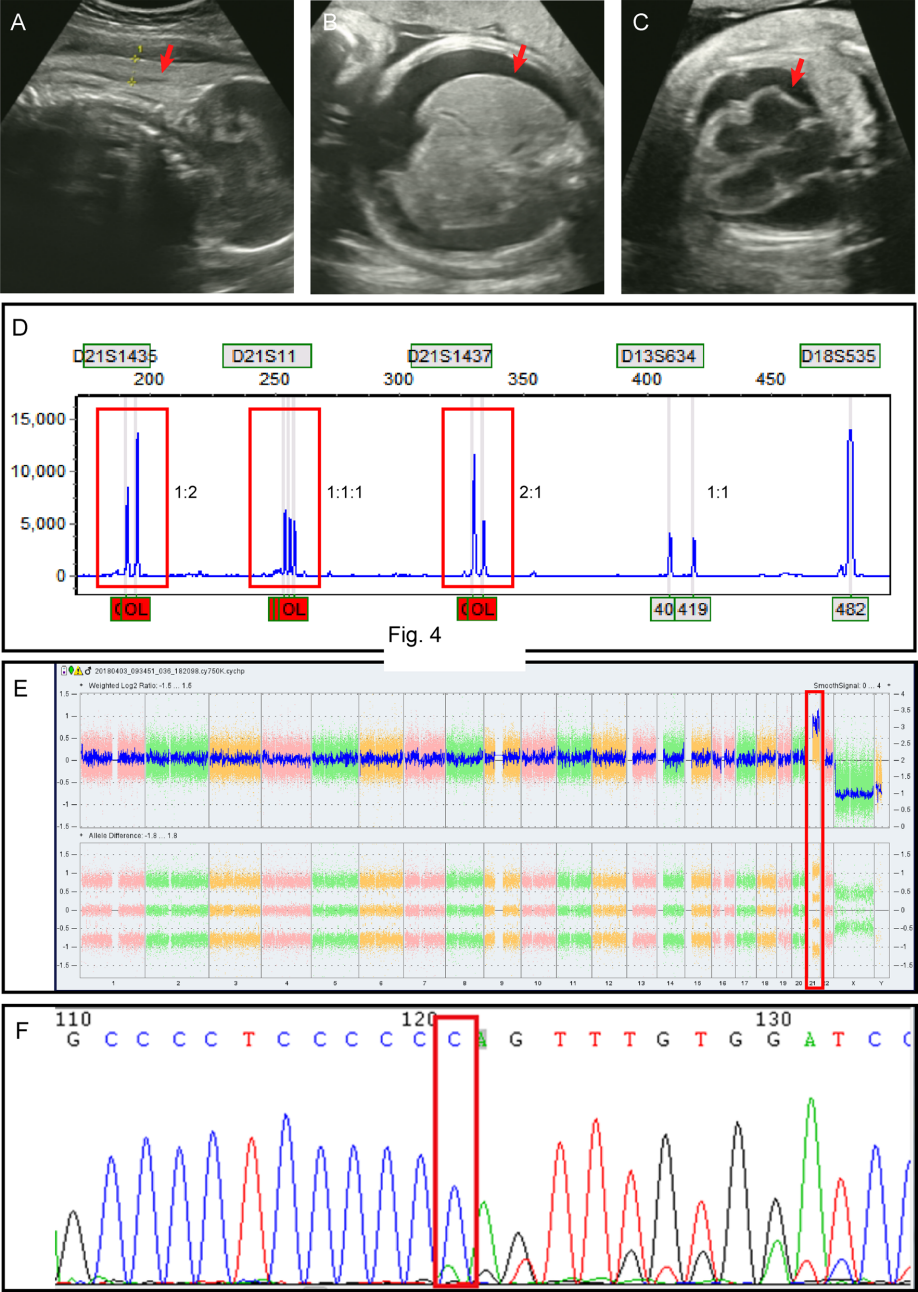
Case2 A–C: The 31-week ultrasound findings of the foetus. A The foetus had thickened skin on the head and chest. B Foetal ascites. C Foetal heart enlargement combined with pericardial effusion. D Electrophoretograms of multiplex QF-PCR amplification with STR markers on chromosomes 18, 13 and 21. Electrophoretic profiles observed for trisomy 21 foetus (red boxes). E Image from the Affymetrix Chromosome Analysis Suite Software showing trisomy 21. The location of the chromosomal repeat segments is denoted by the red boxes. (F) Identification of mutations in *GATA1* by Sanger sequencing, as visualized by Chromas software. The base change is indicated by a red boxes. Mutation [NM_002049.4 c.49dupC(p. Gln17ProfsTer23)] was detected in the foetus
